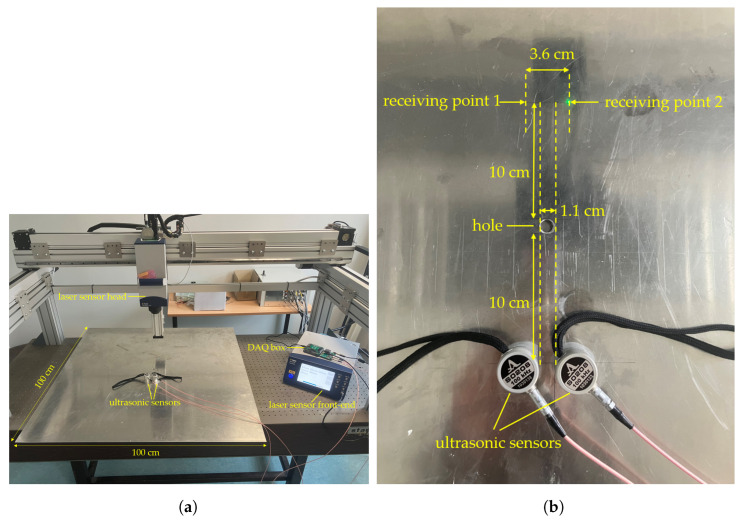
Experimental setup. (a) Measurement setup including ultrasonic sensors, laser vibrometer, and DAQ system. (b) Geometry of the sensors, hole, and receiving positions.
